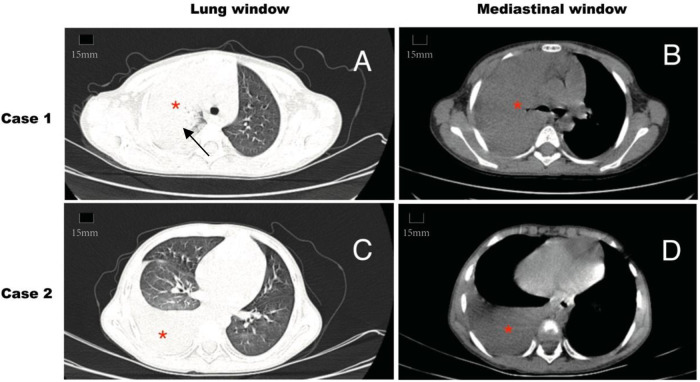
Chest CT manifestations of the two patients. (A) Case 1, lung window: right upper lobe bronchial obstruction (→) and consolidation. (B) Case 1, mediastinal window: massive right pleural effusion (*). (C) Case 2, lung window: right lower lobe consolidation. (D) Case 2, mediastinal window: massive right pleural effusion (*). Scale bars = 15 mm.
